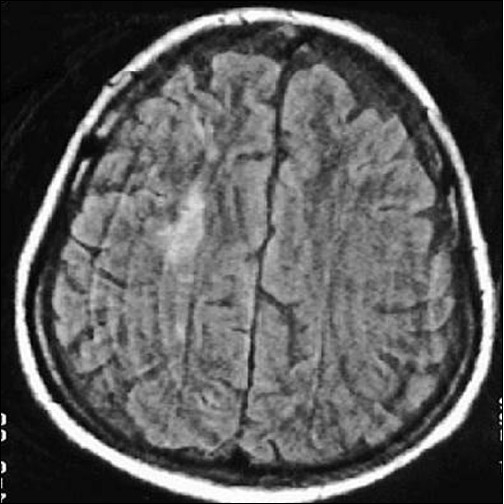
FLAIR sequence shows more intense lesion as compared to initial study (first follow-up study of same patient done 35 days after acute onset)
